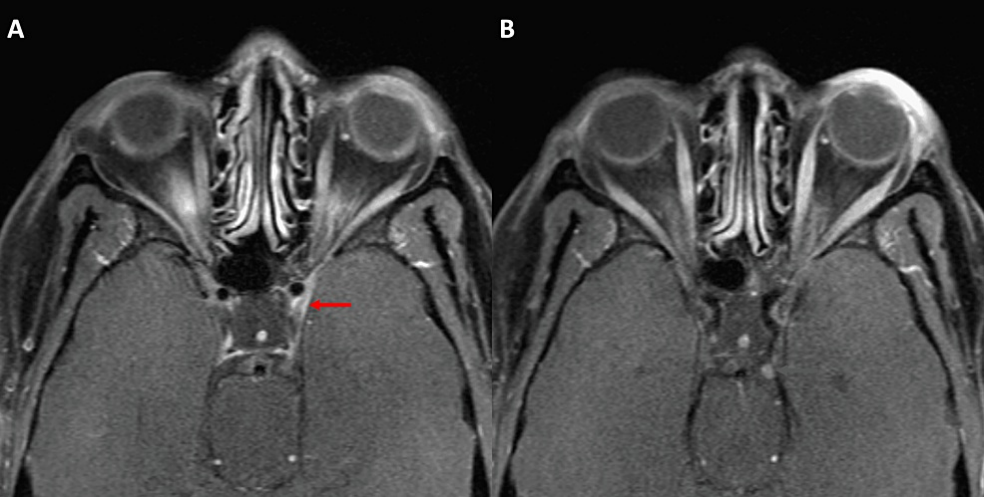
MRI orbit obtained four months later revealed a marked reduction in the thickening of the cavernous segment of the left oculomotor nerve (red arrow in A) and mild reduction in the cisternal segment (green arrow in B).
